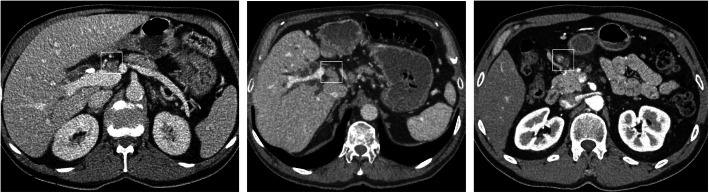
CT images of patients with and without lymph node metastases. a Patient with enlarged suspicious lymph node adjacent to the portal vein and hepatic artery who had no lymph node metastases on pathology. b Patient with enlarged suspicious lymph node adjacent to the hepatic artery who had lymph node metastases on pathology. c Patient with multiple suspicious lymph nodes based on size and inhomogeneity who had lymph node metastases on pathology
